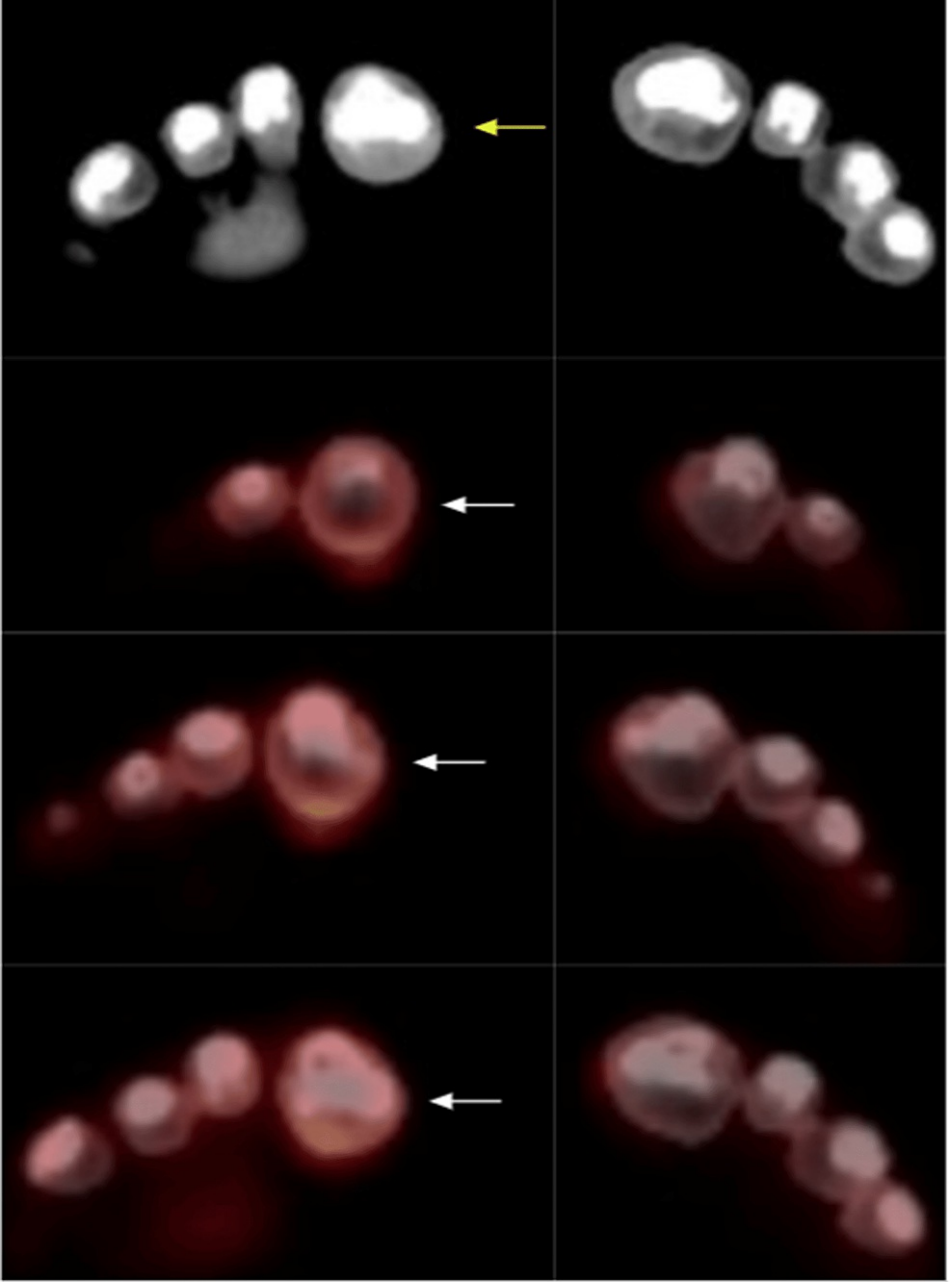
Right first toe focal moderate level increased FDG localization (white arrows) without abnormal anatomical correlate (yellow arrow). FDG: Fluorodeoxyglucose
